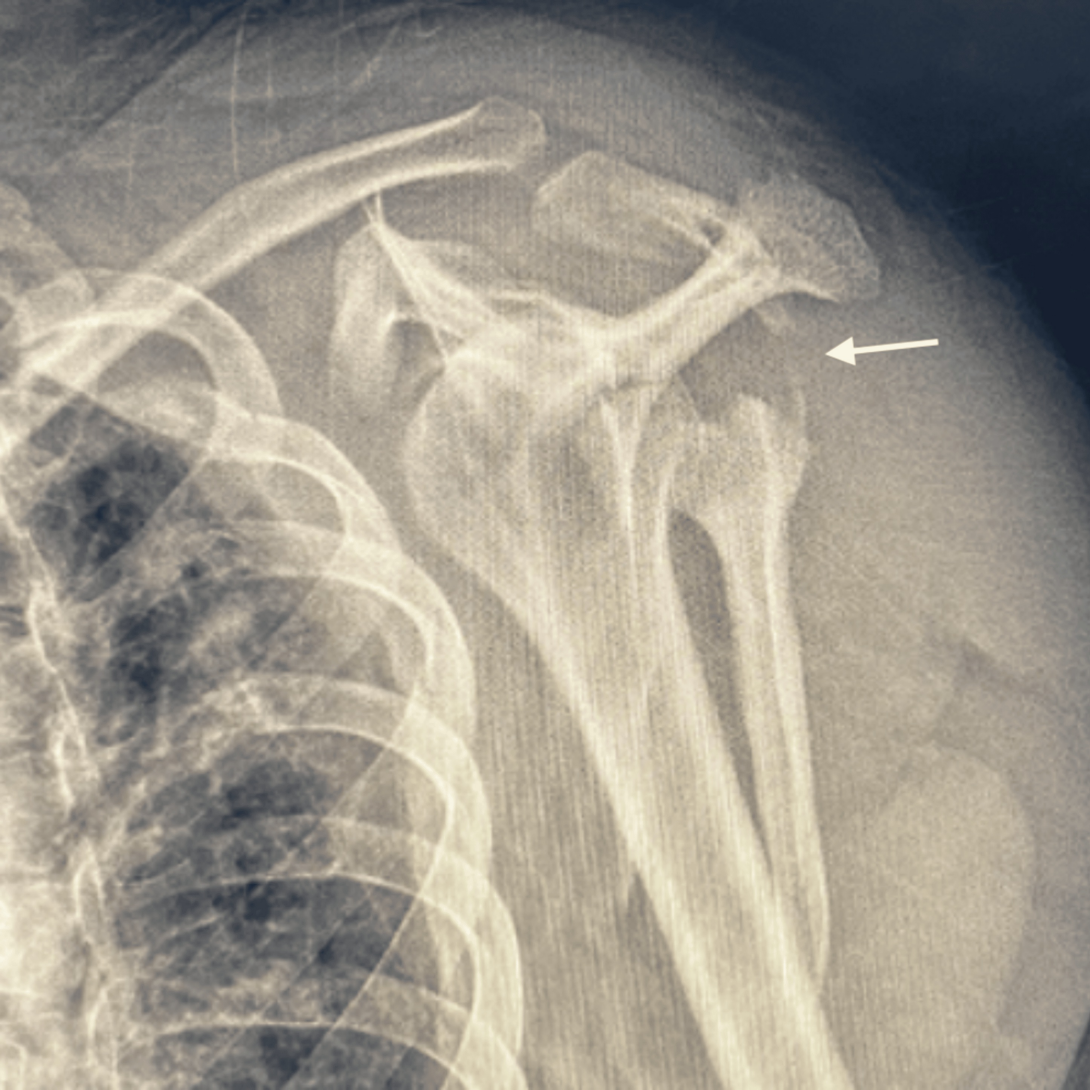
Scapular Y-radiographic view of the left shoulder showing a multifragmentary fracture.
